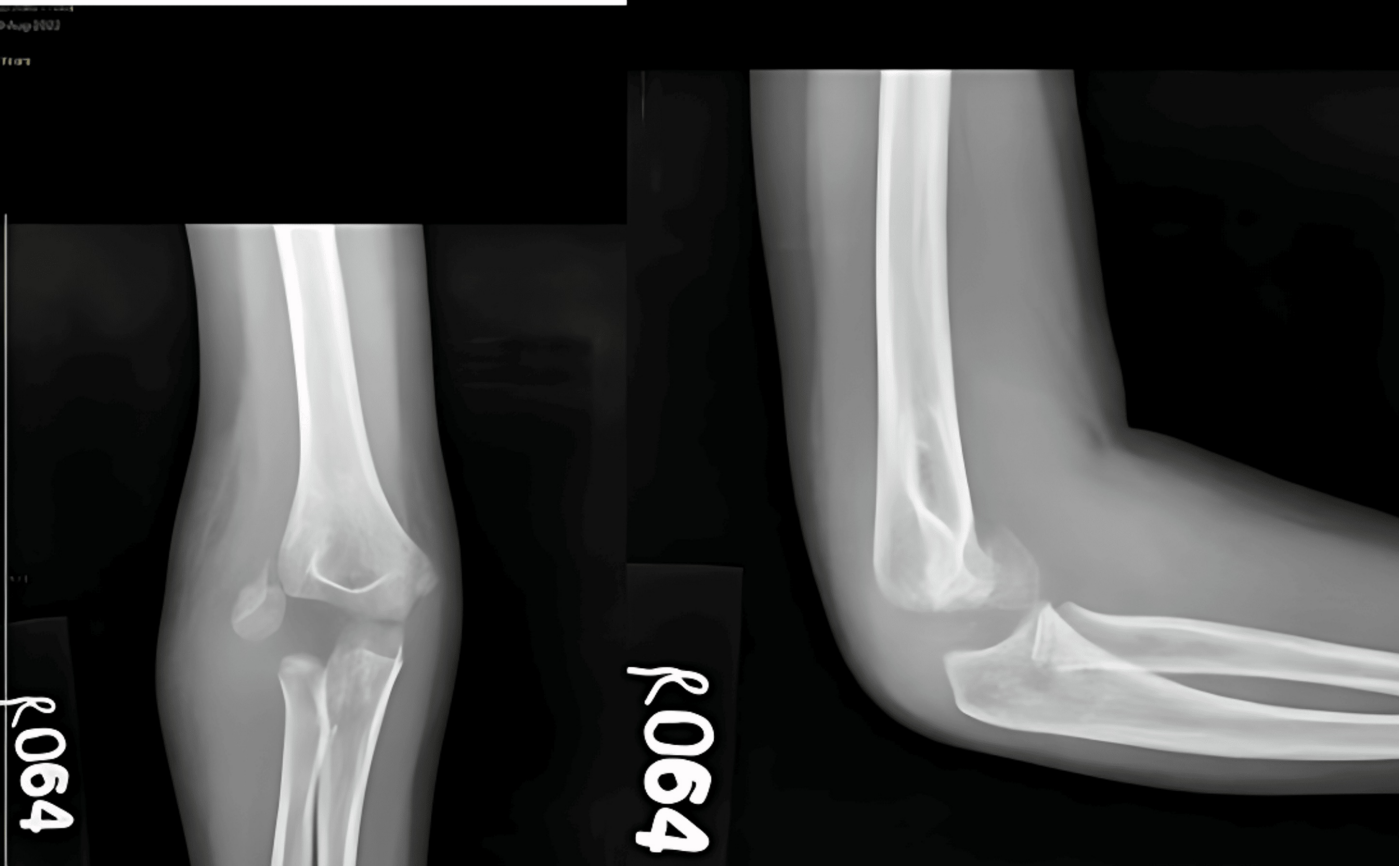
Preoperative X-ray of case 2 showing lateral condyle humerus fracture
